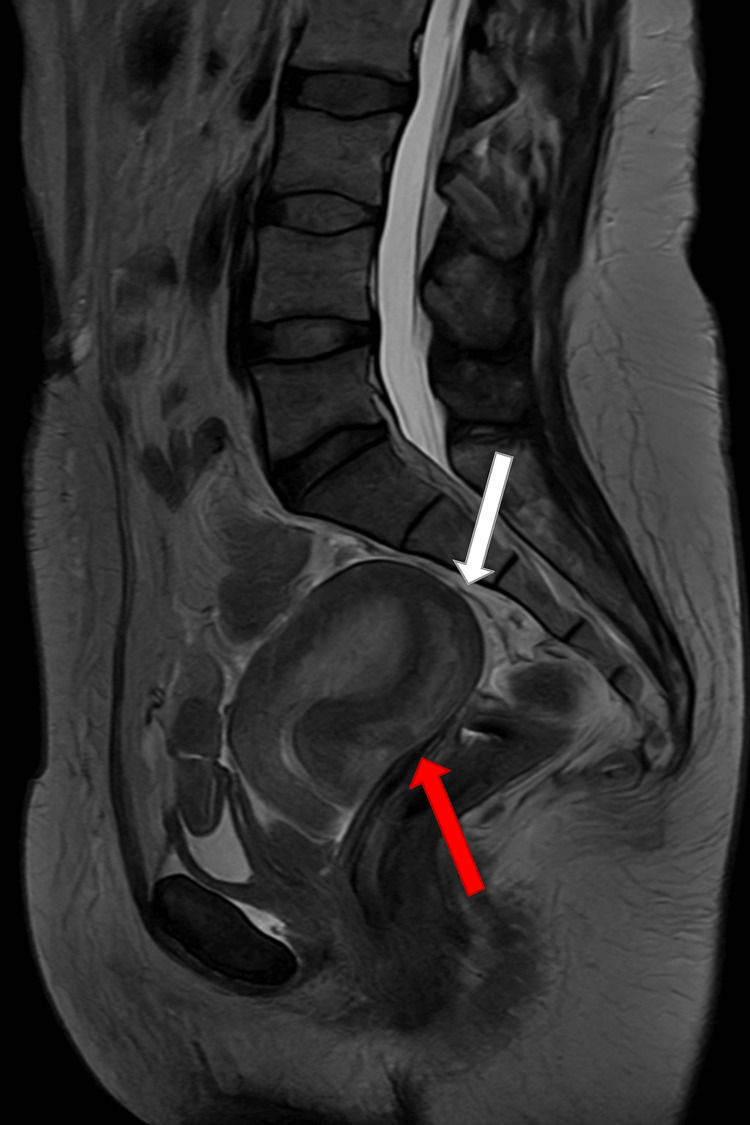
Pelvic MRI (sagittal view) demonstrating rectosigmoid intussusception The bowel-within-bowel configuration is shown. The red arrow highlights the intussusceptum (inner telescoping bowel loop), while the white arrow indicates the intussuscipiens (outer receiving bowel loop).
